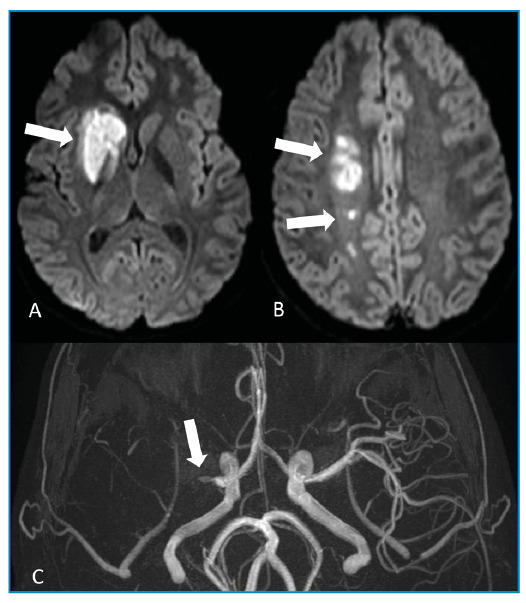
A and B. Axial diffusion-weighted images (DWI) showing right basal ganglia, internal capsule, and deep white matter hyperintensity (arrows), which had corresponding hypointensity on the apparent diffusion coefficient (ADC) map (not shown). This indicates restricted diffusion, consistent with acute ischemic stroke. C. Three-dimensional time-of-flight magnetic resonance angiography (3D-TOF) showing the absence of a flow signal in the right middle cerebral artery starting from its origin (arrow).
